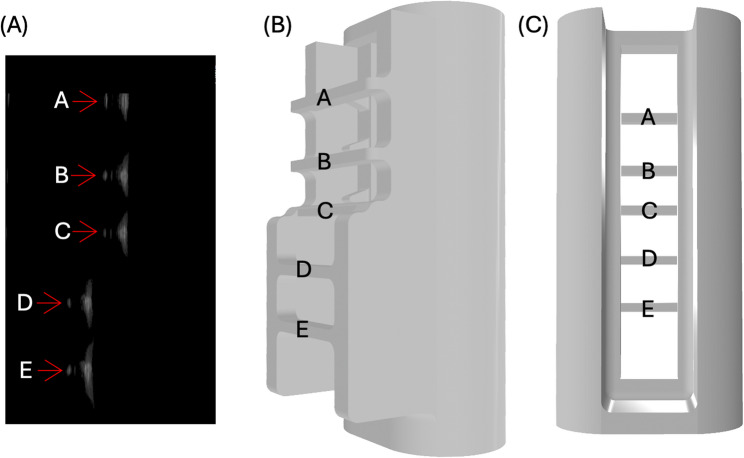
Calibration body with corresponding ultrasound image. A Ultrasound (US) image acquired with the US probe positioned in the calibration body immersed in a water bath. The US markers A–E are visible in the image. B Side view of the calibration body showing the US markers A–E aligned vertically at the heights of measurement points A–E. C Front view of the calibration body
